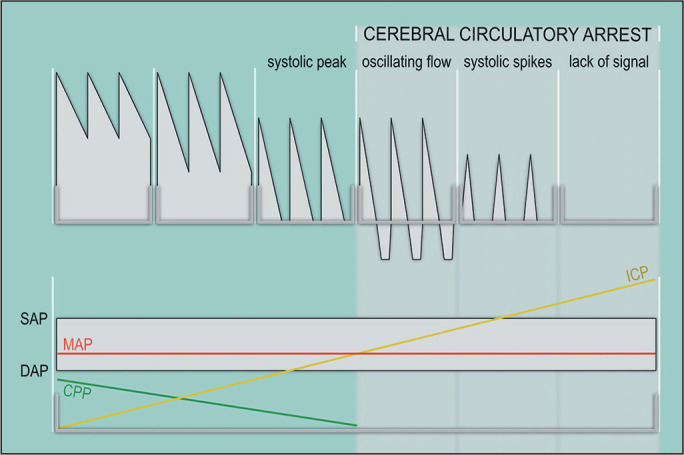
Doppler spectral wave forms from a normal recording to the complete
